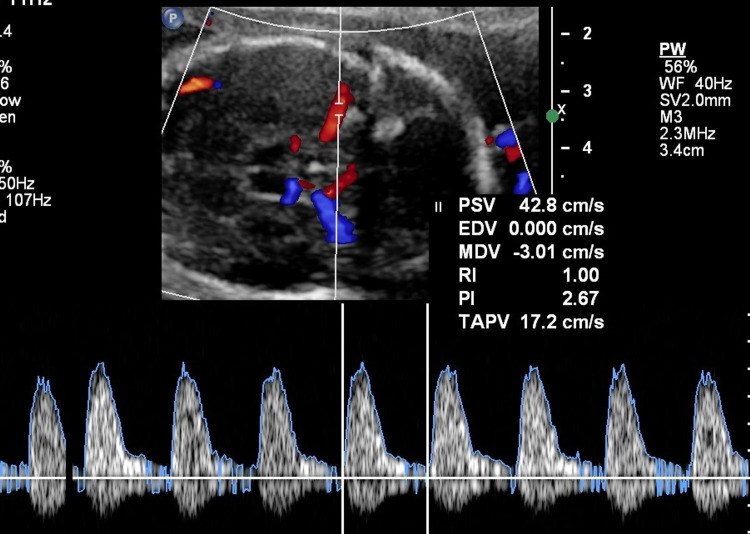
Doppler showing peak systolic velocity of 29.4 (1.60 MoM) MoM: multiple of median
